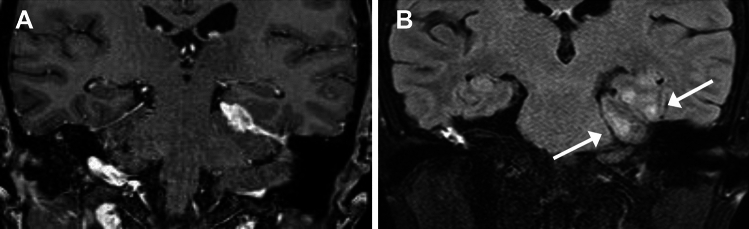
Hypertrophic pachymeningitis (HP) in ANCA-associated vasculitis in a 64-year-old woman with granulomatosis with polyangiitis. (A) Coronal FS-CE-T1WI shows HP involving the left side of the tentorium. (B) FS-FLAIR shows hyperintensity in adjacent brain parenchyma (arrows)
